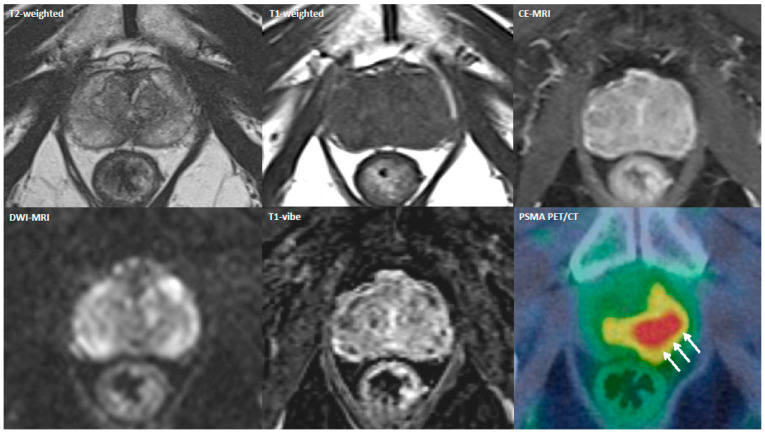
Multipanel comparison between mpMRI (PI-RADS 3) and [^68^Ga]PSMA PET/CT (SUVmax 4.3; SUVratio to background 2.1) in a biopsy-naïve patient with PSA of 5.54 ng/mL. The highlighted lesion resulted in a GS 3+4 adenocarcinoma of the left prostatic lobe (white arrows). PSMA = prostate-specific membrane antigen; PET/CT = positron emission tomography/computed tomography; CE − MRI = contrast − enhanced MRI; DWI − MRI = diffusion − weighted imaging MRI.
